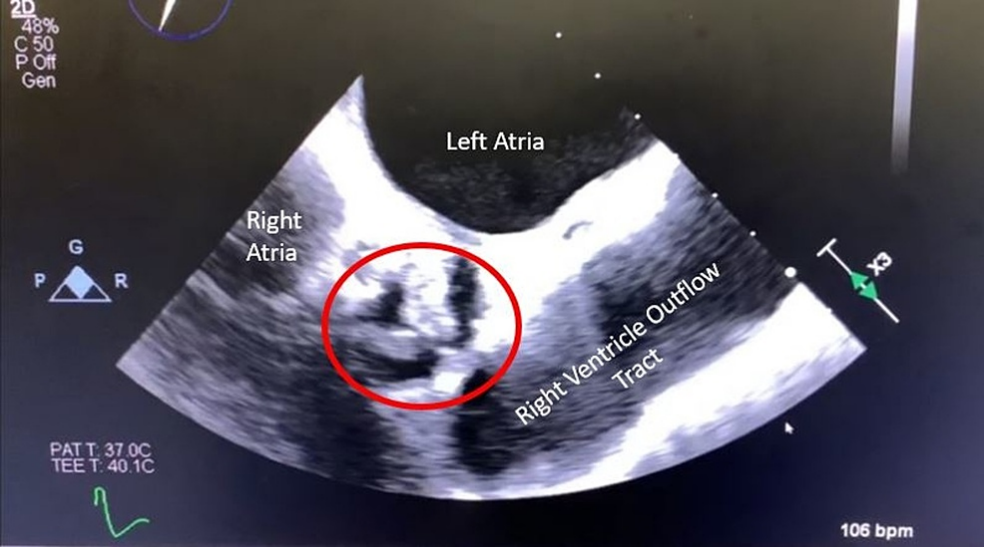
Transesophageal echocardiogram short-axis view with aortic valve vegetation circumscribed
